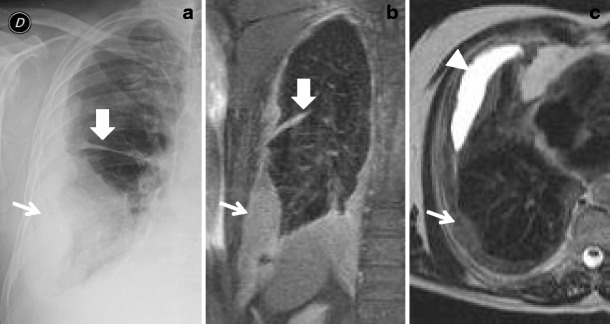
Biphasic mesothelioma; chest X-ray film (a) showing a right basal and lateral pleural thickening with fissure involvement (*thick arrow*). Lung MR confirm these findings, both on fat saturation T1-weighted image (b) and spin echo T2-weighted sequence (c) that better demonstrates a mild basal anterior pleural effusion (*arrowhead*)
